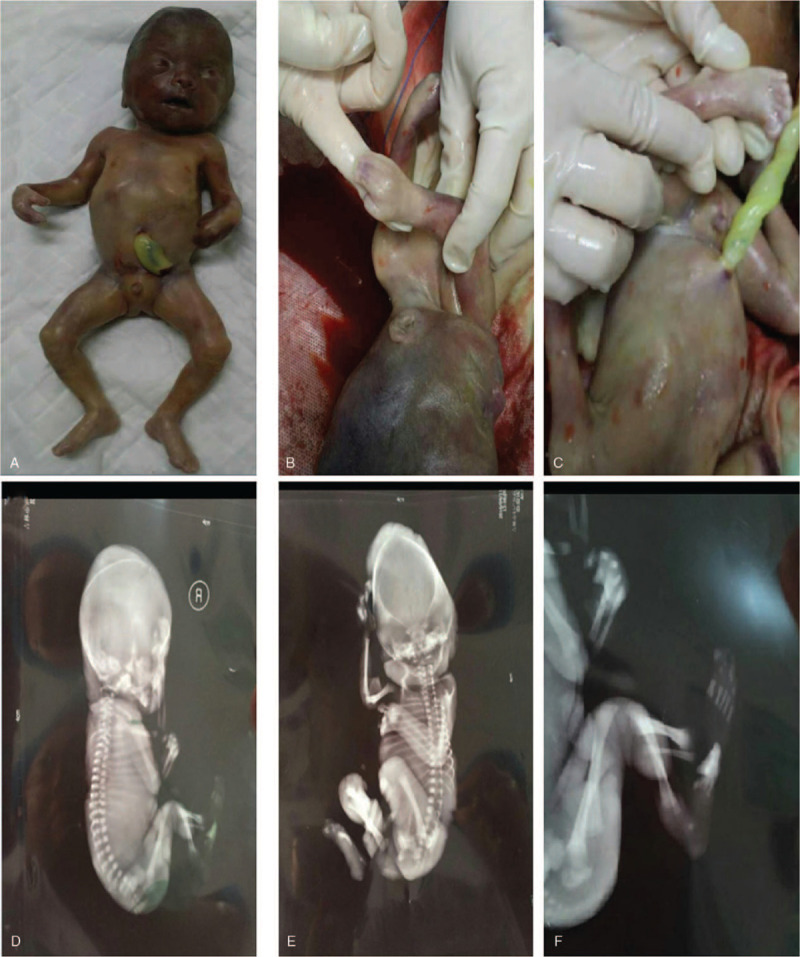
The dead fetus after induced labor. (A–C) Physical characteristics of acrocephalosyndactyly. (D–F) Presentation of acrocephalia as shown by X-ray. Craniosynostosis was not evident.
